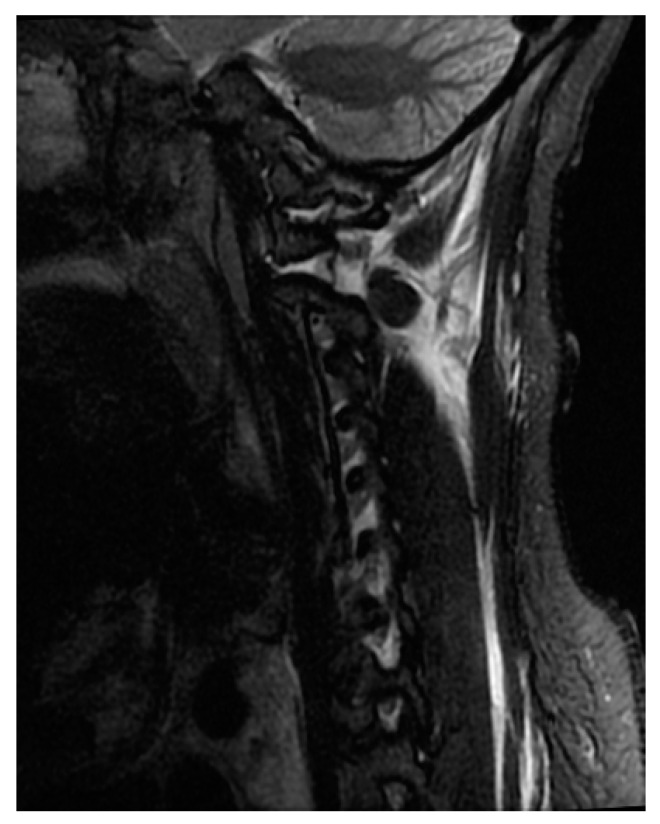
Sagittal view of STIR sequence of MRI shows signal changes in the C1/2 and C1/condyle joint.
